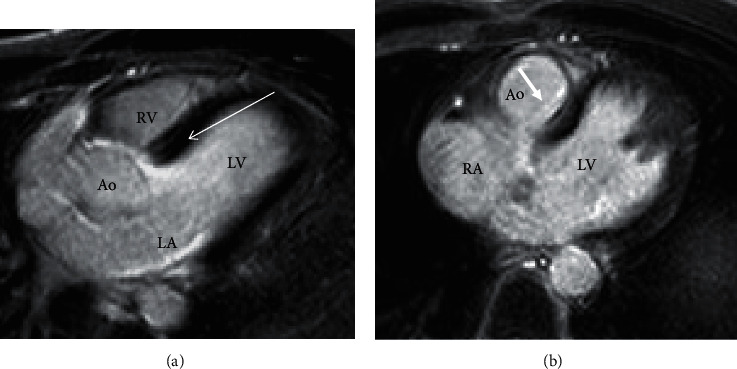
(a) and (b) Two males, aged 42 (a) and 48 (b) years, with idiopathic LBBB (a and b). In both cases, MRI shows late enhancement in the left posterior septum (arrows), possibly due to fibrosis involving the left bundle. Ao = aorta, RV and LV = right and left ventricles, respectively, RA and LA = right and left atria, respectively.
